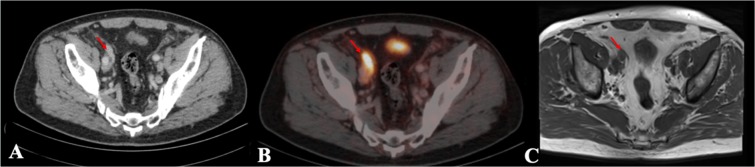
Example of a lymph node larger than 10 mm in ^68^Gallium-Prostate specific membrane antigen PET-CT compared to T1 MRI. This figure shows a suspicious lymph node larger than 10 mm diameter. The lymph node is visualized in corresponding axial plane slices using CT, ^68^Gallium-Prostate specific membrane antigen PET-CT and T1 MRI from left to right. The lymph node is located at the right external artery. The lymph node is highlighted through red arrows. (A) Lymph node depicted in CT, (B) Lymph node depicted in ^68^Gallium-Prostate specific membrane antigen PET-CT, (C) Lymph node depicted in the T1 sequence in MRI.
